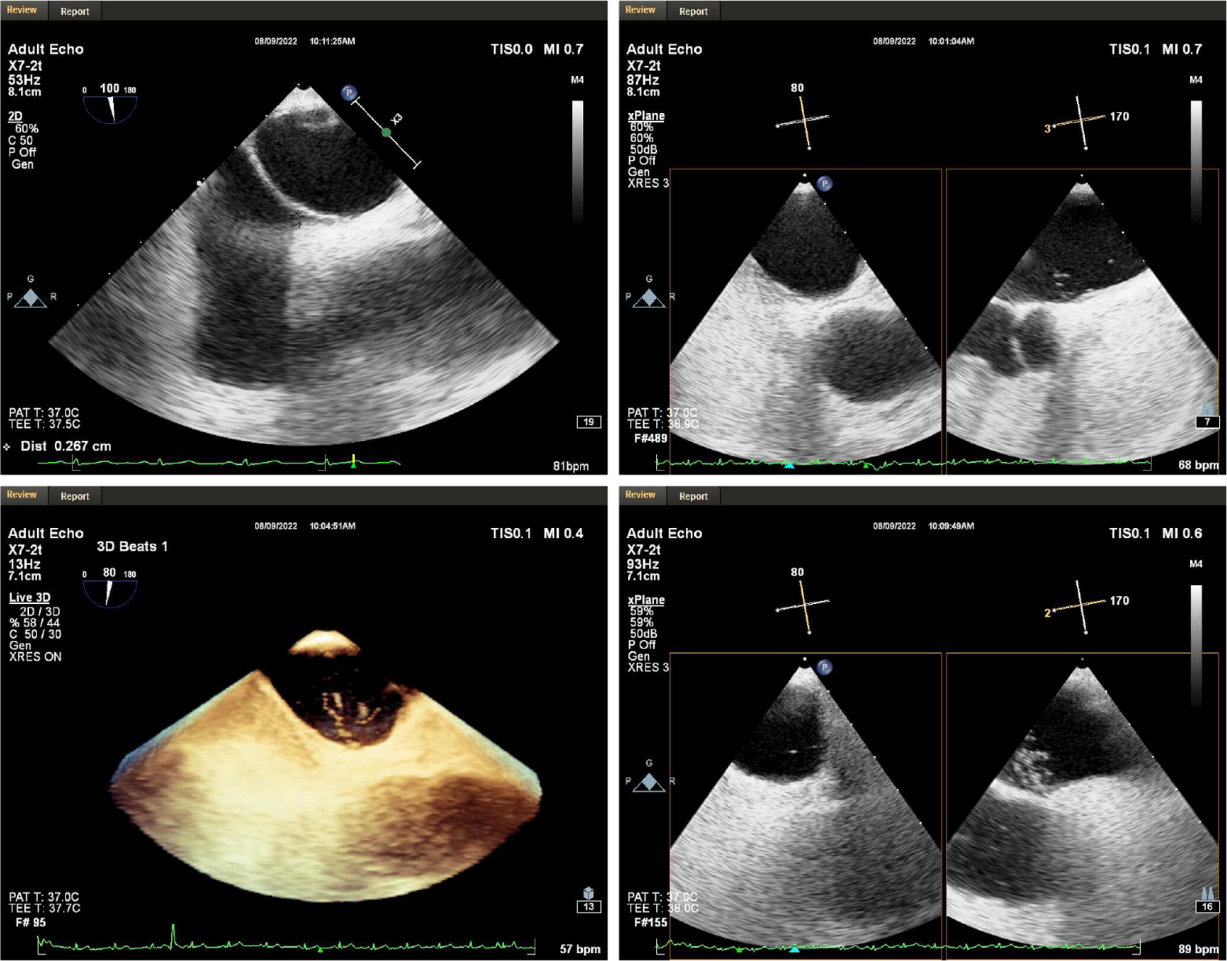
Transesophageal echocardiography. A PFO with a length of 9.7 mm, a diameter of 2.7 mm in the right atrium and 1.1 mm in the left atrium was observed in the middle of the patient's atrial septum. At rest, a moderate amount of shunt was seen in the left atrium, and a large number of shunt was seen immediately after increasing abdominal pressure.
